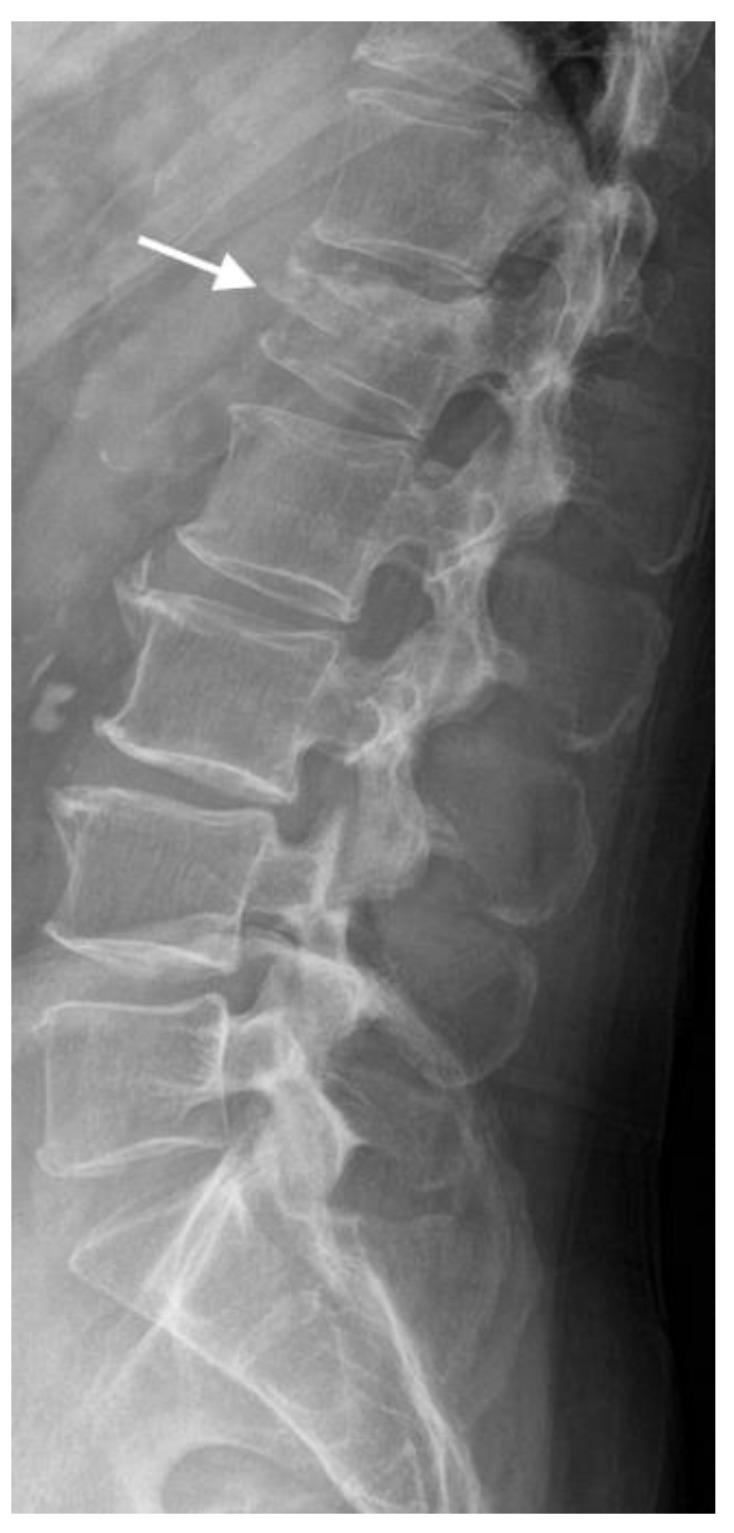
In this lateral radiograph of the lumbar spine, the arrow demonstrates compression fracture of the superior endplate of L1 with mild to moderate vertebral height loss.
